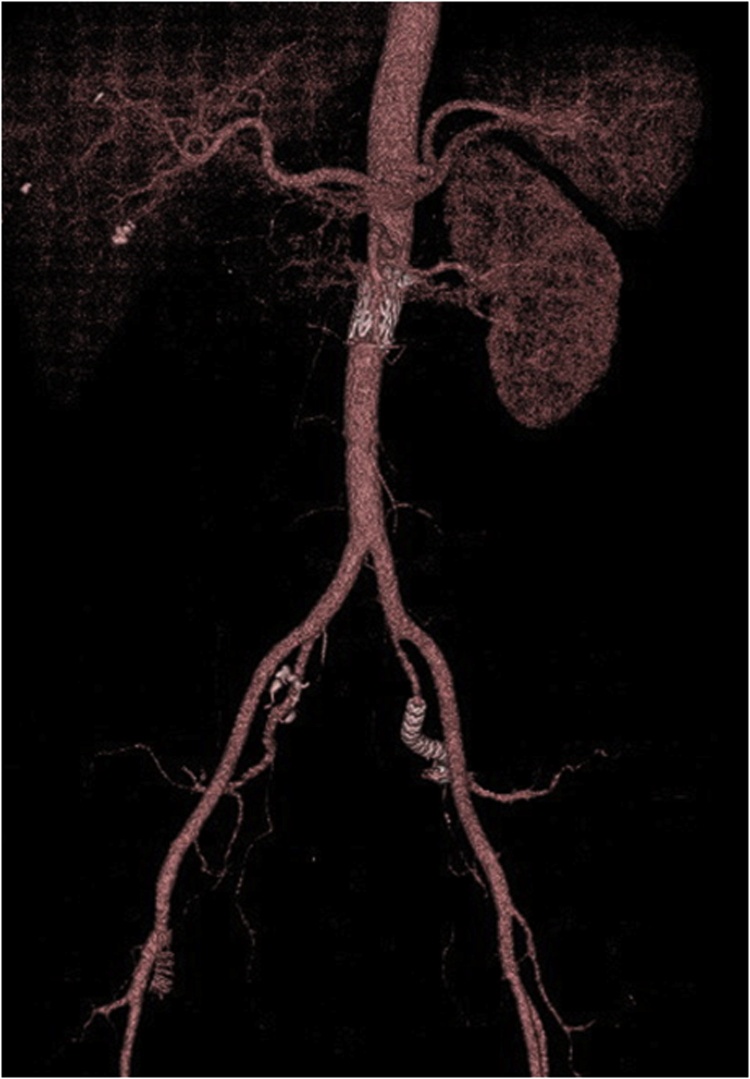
Postoperative 3D computed tomography. No endoleak was observed, and the right renal artery was not enhanced.
